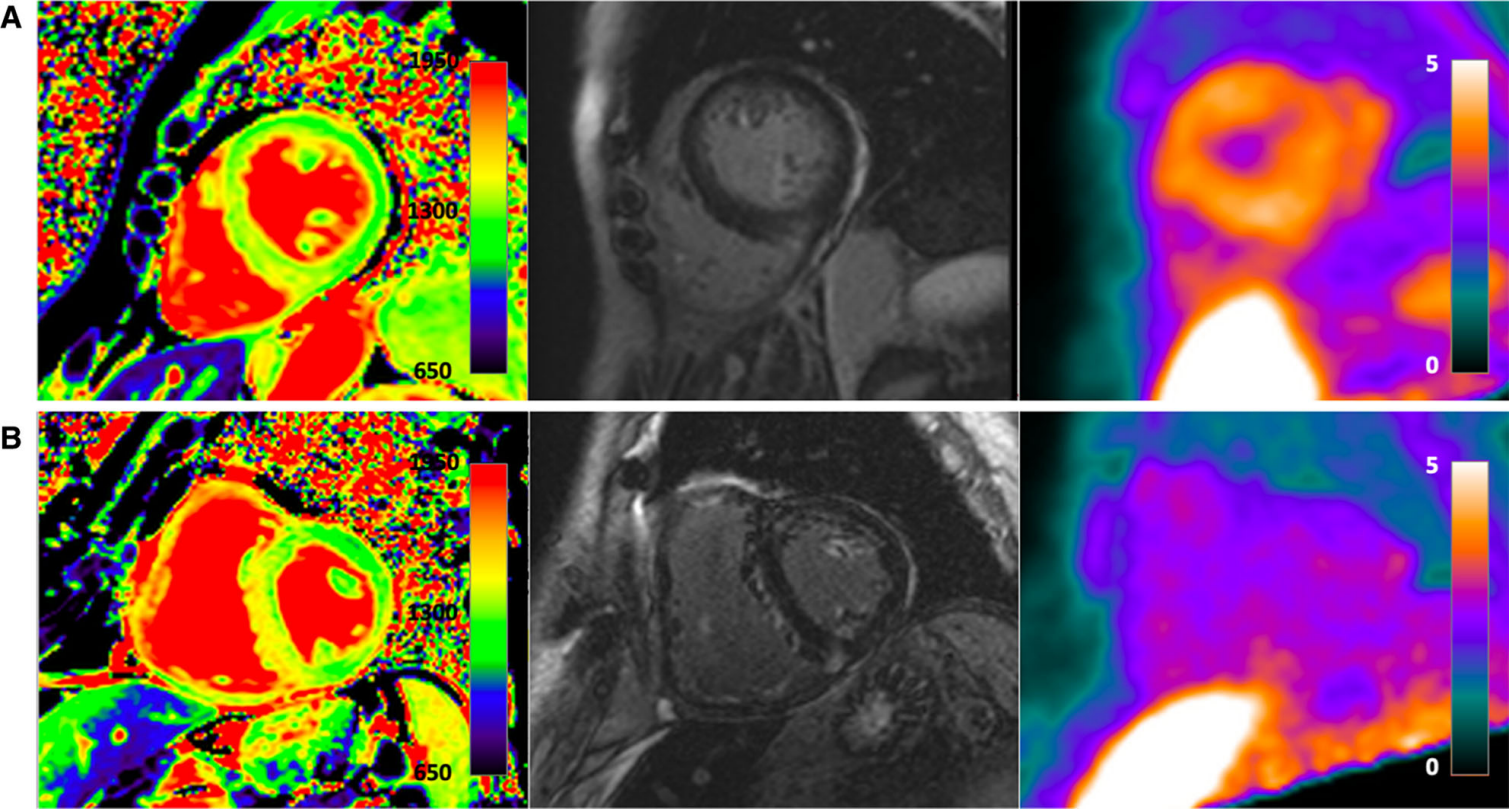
^18^F-flutemetamol PET/MRIs of a PET-positive, female patient with hereditary ATTR-CA (A) and a PET-negative male patient with wild-type ATTR-CA (B). Representative short-axis images of native T1 maps (left column), late gadolinium enhancement (middle column), and F-18 flutemetamol PET (right column). While patient A demonstrates an abnormal T1 map (1448 ms), diffused LGE, and an increased tracer uptake (SUV_mean_ myocardium: 3.1, SUV_mean_ blood pool: 1.3), patient B does not demonstrate an increased tracer uptake (SUV_mean_ myocardium 1.4, SUV_mean_ blood pool: 1.5) despite an abnormal native T1 map (1460 ms), focal areas of LGE, and a positive myocardial biopsy and bone scan (Perugini 3, not shown)
